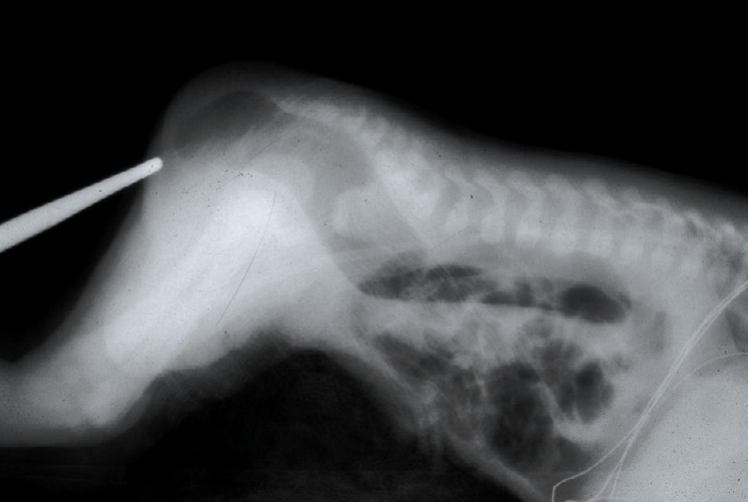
X-ray, cross-table lateral film with the baby in prone position.
